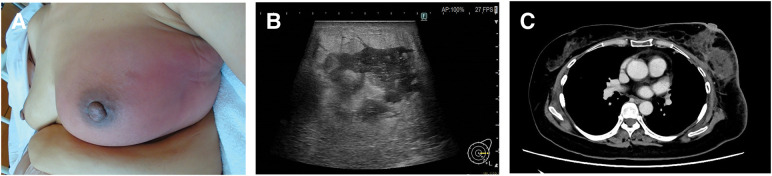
Clinical and imaging findings (A) Despite antibiotic therapy, the left breast exhibited marked swelling, erythema, and warmth. Prominent swelling was observed at the RFA-treated site in the C area. (B) Ultrasonography revealed fluid collection at the RFA site, suggesting abscess formation. The surrounding subcutaneous tissue exhibited inflammatory edematous changes. (C) Contrast-enhanced CT demonstrated fluid collection consistent with abscess formation centered at the RFA ablation site. RFA, radiofrequency ablation
